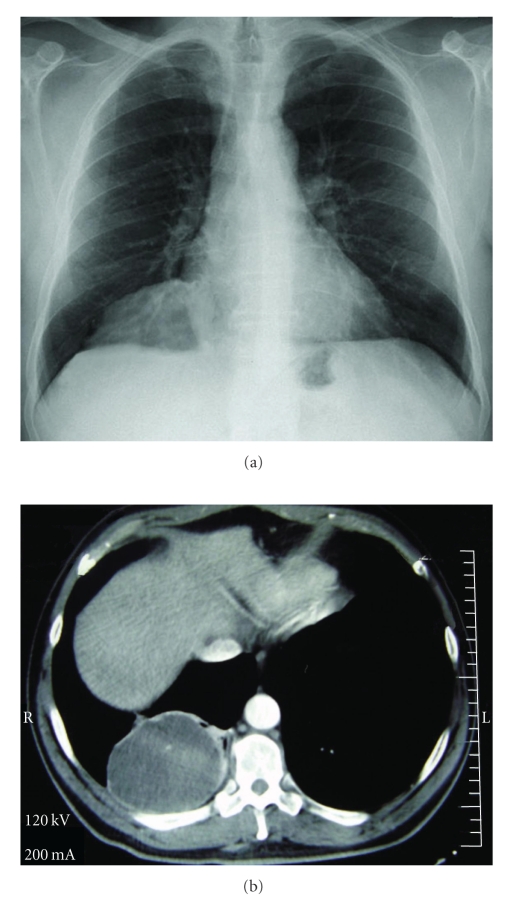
Primary PMCAC. (a) Chest X-ray revealing a solitary tumor in the right lower lobe of the lung. (b) Computed Tomography scan of the thorax. A large cystic well-demarcated mass in the right lower lobe of the lung is detected (19 Hounsfield Units).
